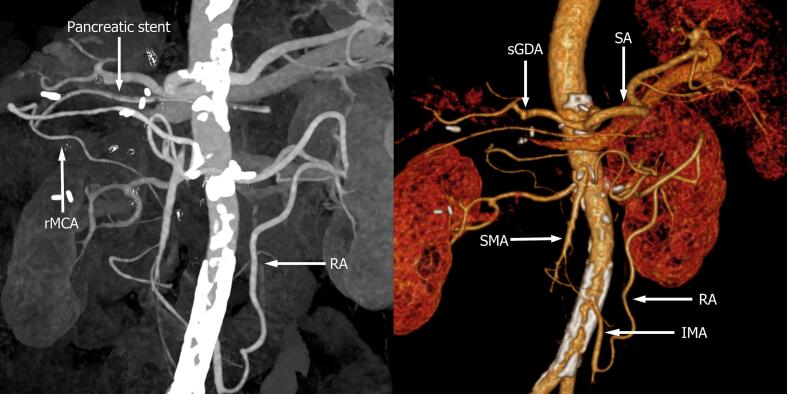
Postoperative CT-reconstruction of arterial blood supply. Development of Riolan's arcade (RA) on POD 32. Dilation of middle colic artery branches. rMCA: right middle colic artery; sGDA: gastroduodenal artery stump;
